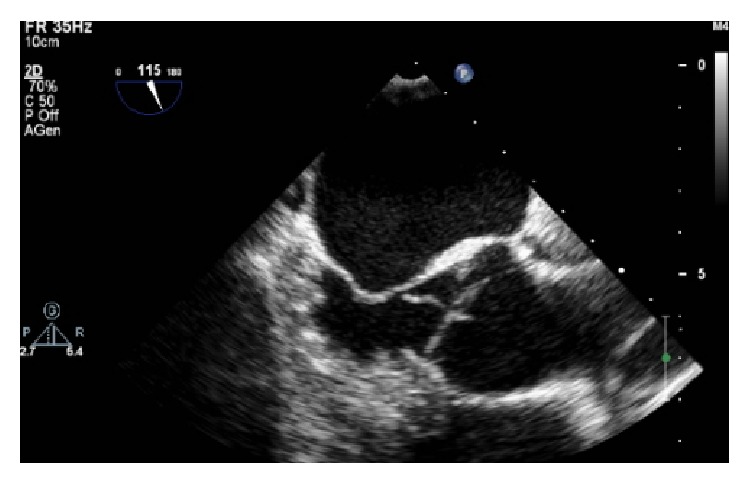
Transesophageal echocardiography. A linear structure arises from the line of closure of the aortic valve.
